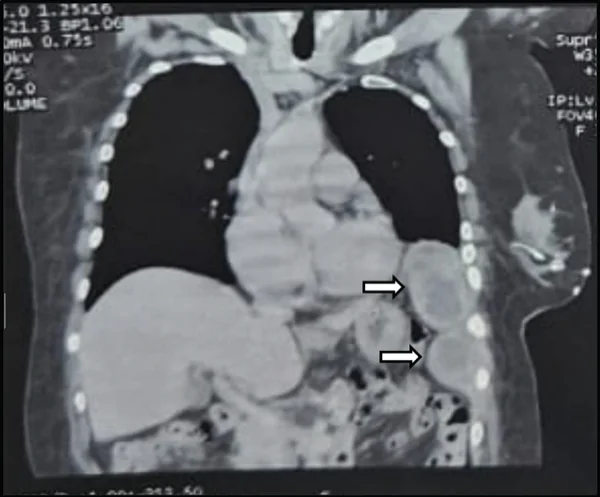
Follow‐up chest CT scan showing biopsy‐confirmed local recurrence in the left hemithorax near the previous surgical field.
